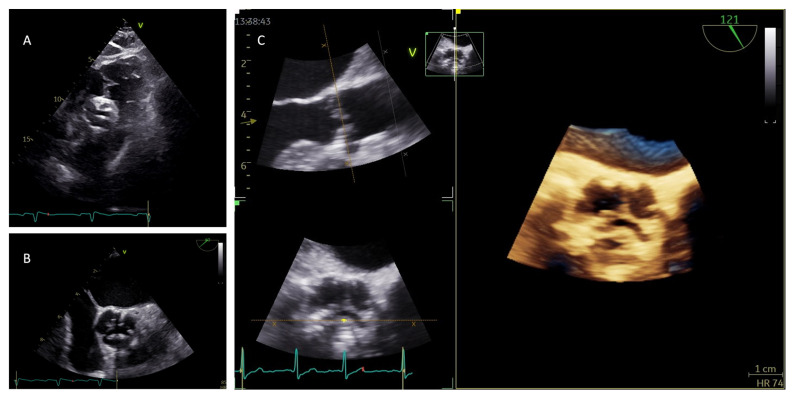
Evaluation of aortic valve morphology. (A) Subcostal view with 2D transthoracic echocardiography might provide a valid alternative in the absence of an adequate parasternal short axis view. (B) Short axis view with 2D transesophageal echocardiography. (C) 3D transesophageal echocardiography for the identification of the smallest aortic valve area.
